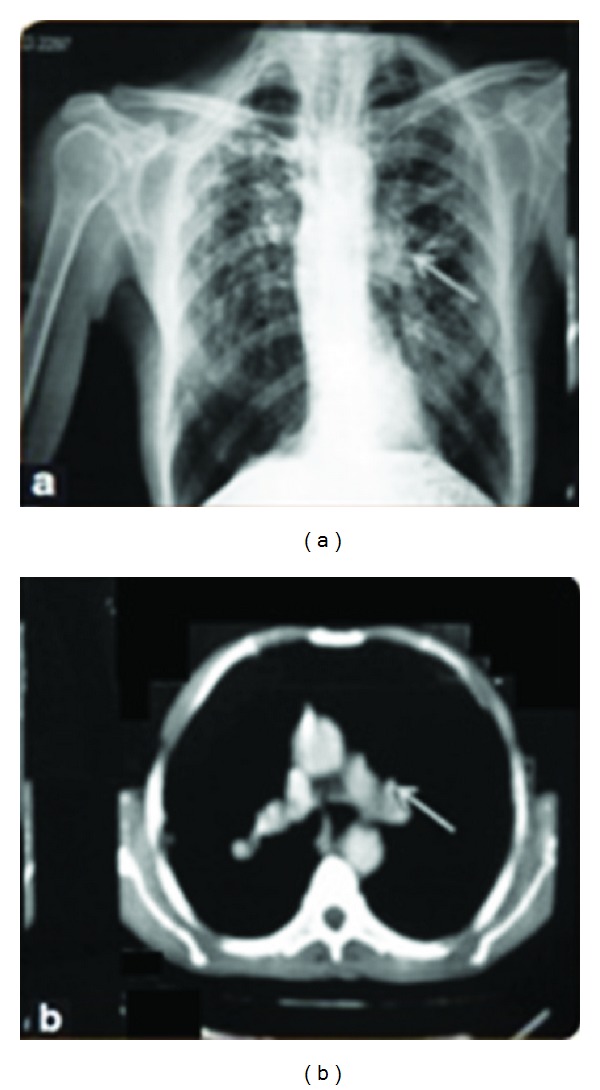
Difference between an X-ray and a CT image, (a) X-ray and (b) CT image.
